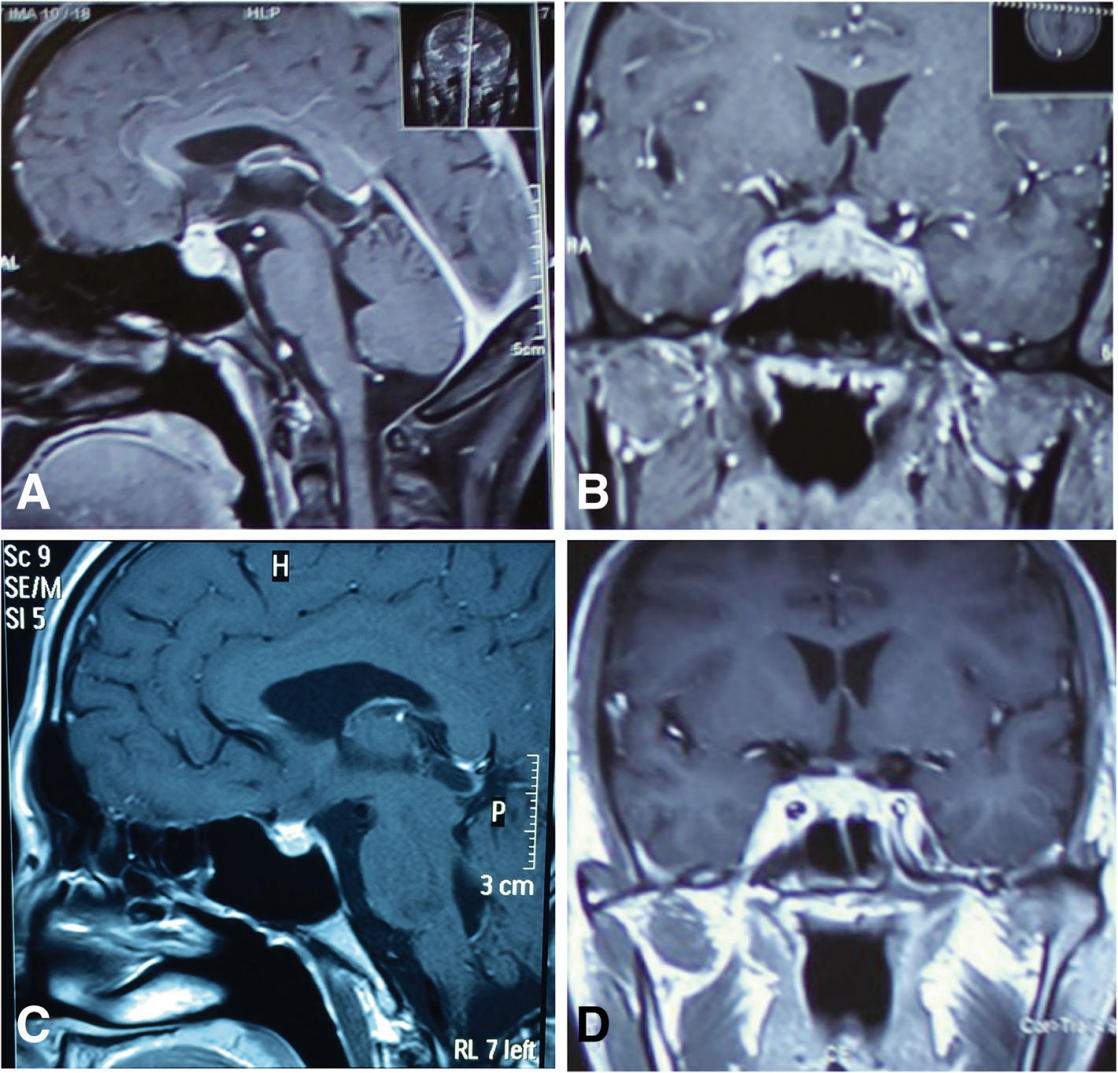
Magnetic resonance imaging (MRI) scans from the first episode: a Enhanced sagittal T1 sequence: enlarged pituitary fossa with a lesion convex to the suprasellar area. The pituitary stalk was compressed. The size of the lesion was 43*15*17 mm, with a slightly long T1 and long T2 signal mixed with a short T1 signal and a long T2 signal with inhomogeneous enhancement. No obvious bone destruction was detected. b Enhanced coronal T1 sequence: optic chiasm compression with the bilateral cavernous sinus surrounded by the lesion. c Enhanced sagittal T1 sequence: the volume of the lesion was reduced significantly after IV glucocorticoid treatment. d Enhanced coronal T1 sequence: the morphology of the optic chiasm and cavernous sinus returned to normal after IV glucocorticoid administration
